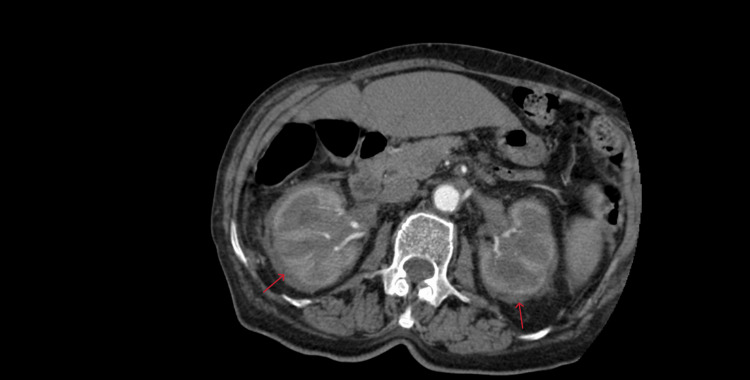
computed tomography scan of the kidneys (axial view) CT showing bilateral perirenal soft tissue halo.
